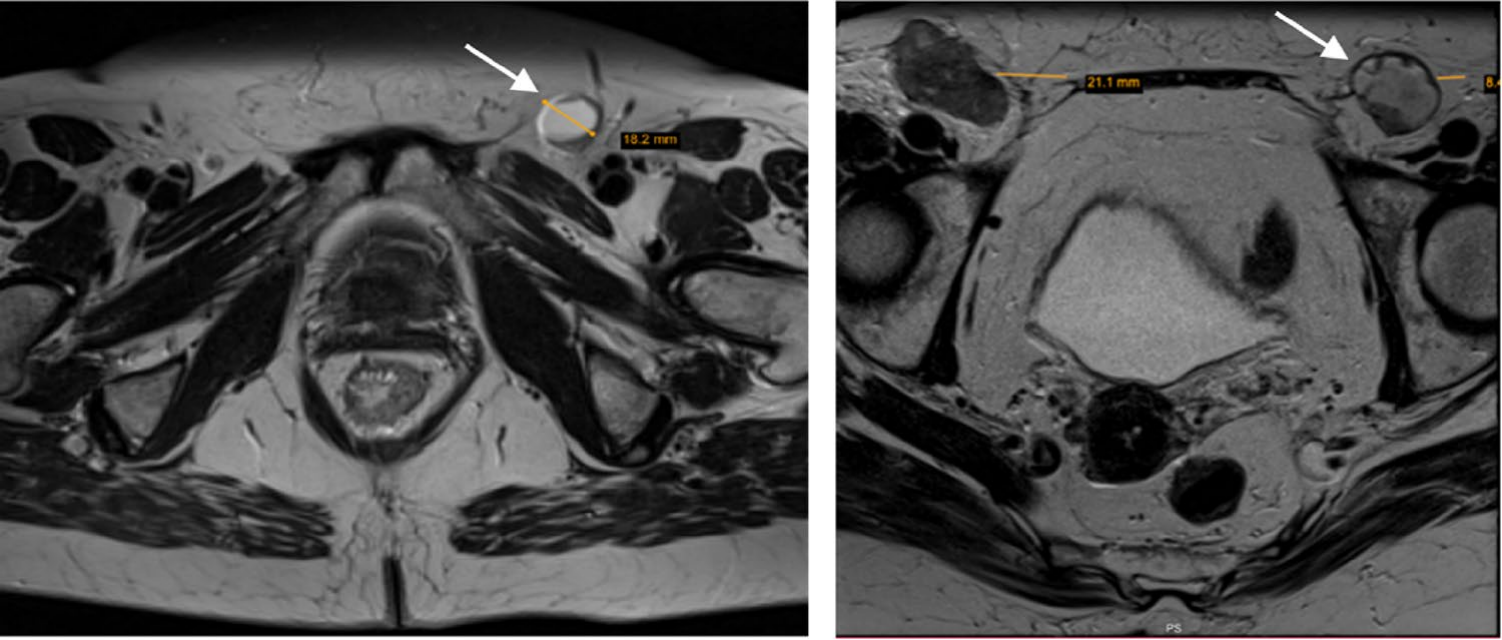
Two examples of PET-CT-positive left inguinal lymph nodes with large central necrosis on T2-weighted MR-image (white arrows)
